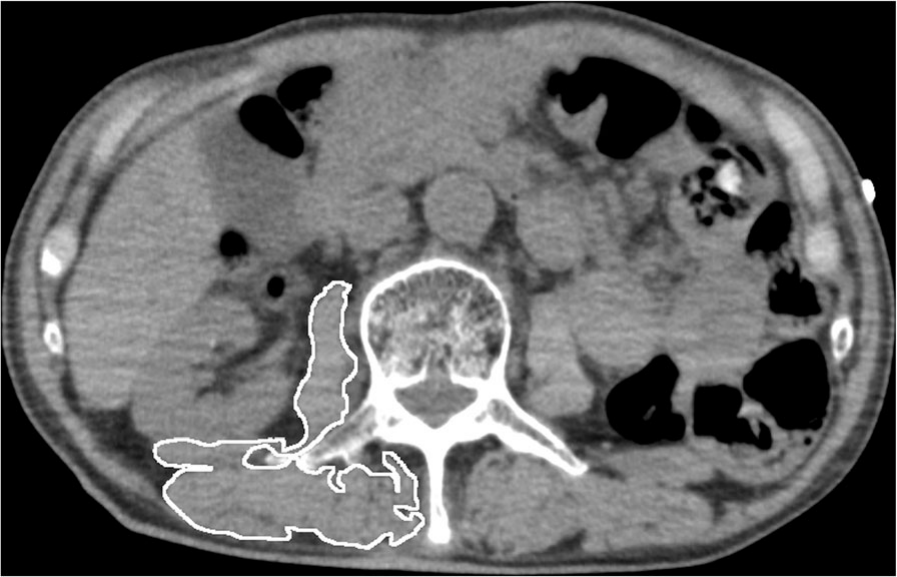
CT image at the L3-sectional muscle. The right L3-sectional muscle area is outlined. Muscle area and mean muscle attenuation are calculated by the picture archiving and communication system (PACS) software
